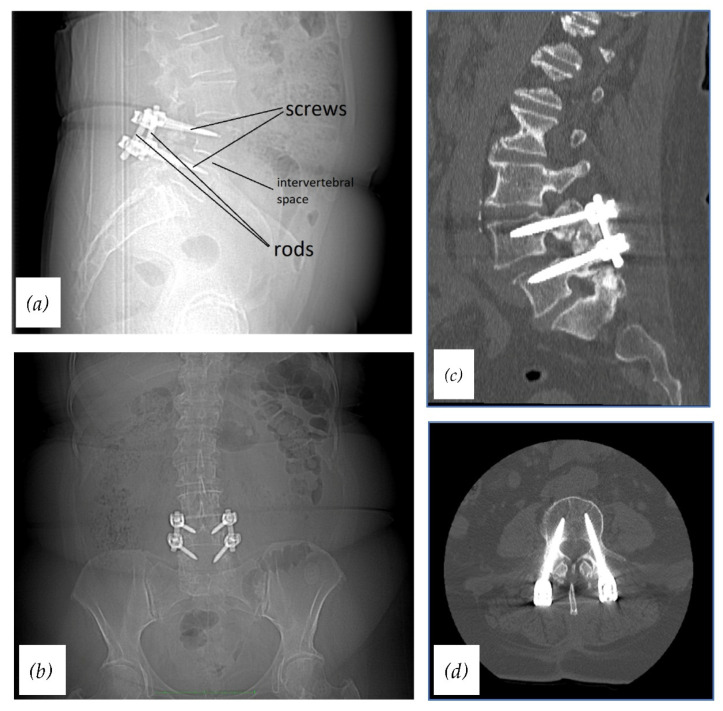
Radiographs and CTs of stabilized lumbar spine using the standard spinal fixation device: (a) Radiograph, lateral view (with descriptions); (b) radiograph, AP view; (c) CT, sagittal plane; (d) CT, axial plane.
